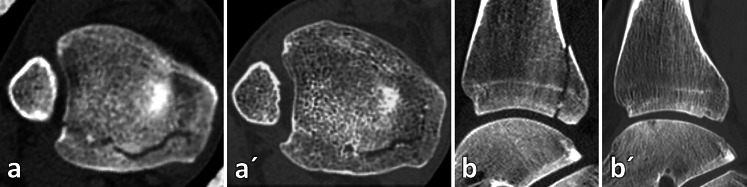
Patient N-3 – widening of the tibiofibular space at the final follow-up. a – an axial postinjury CT scan shows widening of the tibiofibular space of 1 mm and a fracture of PM type 3 according B-R classification; a´ - an axial follow-up CT scan 41 months after injury, documenting widening of the tibiofibular space to 2 mm; b – a sagittal postinjury CT scan with 1 mm proximal displacement of PM; b´ - a sagittal follow-up CT scan 41 months after injury shows healing of PM with less than a 2 mm displacement
